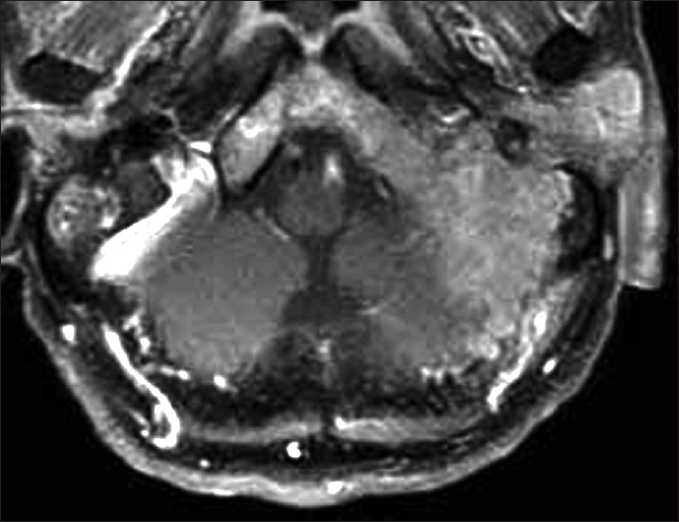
Gadolinium-enhaced T1-weighted MR image of the brain demonstrating an enhancing mass with diffuse infiltration into the clivus and petrous bone
